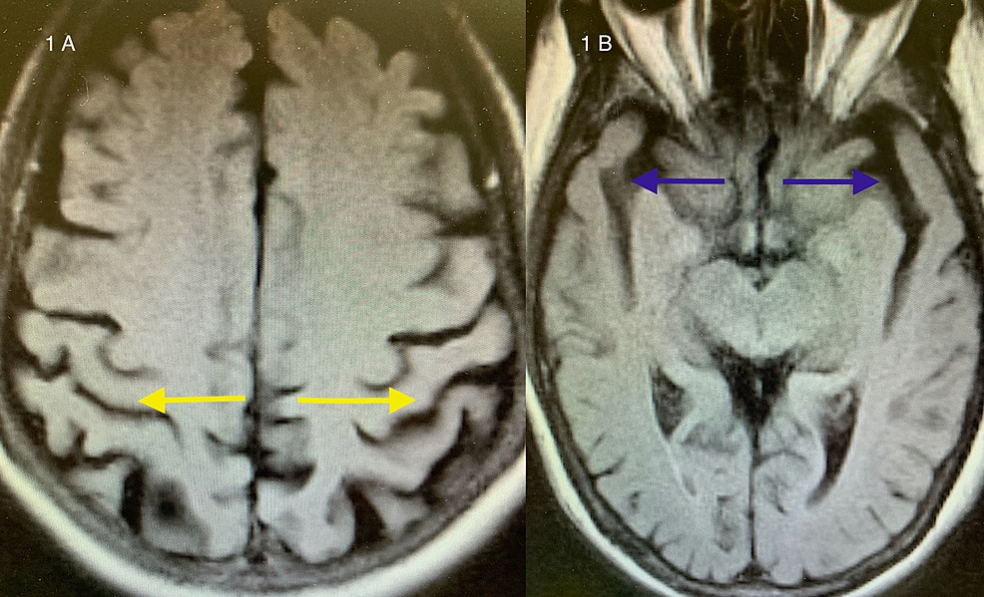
Axial FLAIR MRI of the brain 1A: biparietal lobe atrophy (yellow arrows); 1B: bitemporal lobe atrophy (blue arrows) FLAIR: fluid-attenuated inversion recovery; MRI: magnetic resonance imaging
